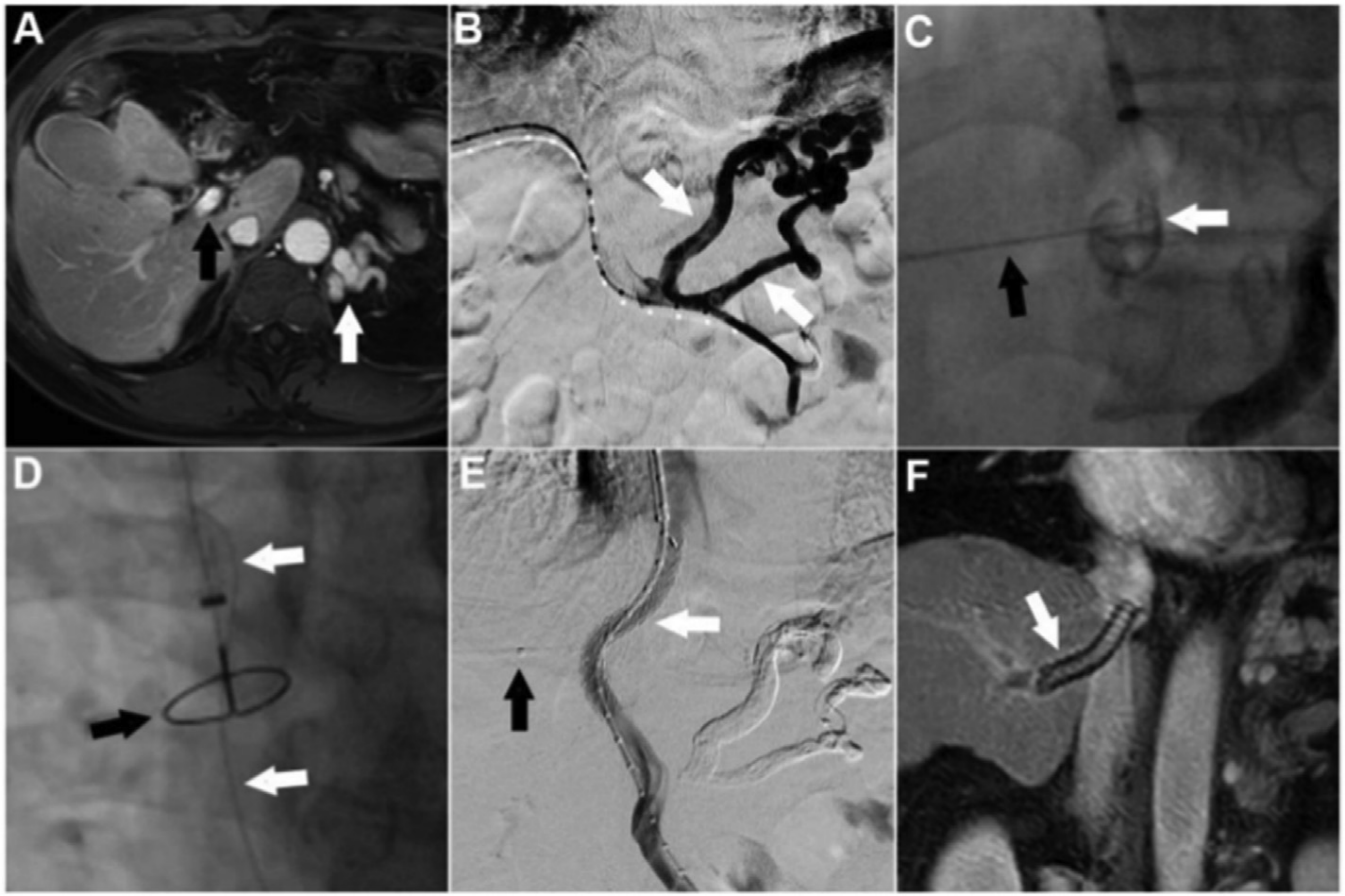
Number four patient: A) The patient showed severe atrophy of the left hepatic lobe and left portal vein after hepatectomy and TACE therapy, and the portal vein completely exposed outside the liver parenchyma (black arrow). B) Two gastric varices (white arrow) with gastrorenal shunts was confirmed by transhepatic portography. C) A 21-gauge, 15-cm needle (black arrow) was used to puncture the gold-marked catheter (white arrow) via a 6-Fr sheath in the right portal vein. D) Transhepatic puncture from right portal vein to IVC was performed successfully and the exchange guidewire (white arrow) was captured by a snare (black arrow). E) A Viatorr stent (8 ​× ​60 mm, 20 mm) was inserted and post-stent venography was performed. The 6F catheter (black arrow) was removed after the transhepatic puncture tract was embolized with 14mm-8cm coils. F) MRI scan 24 months after TIPS.
